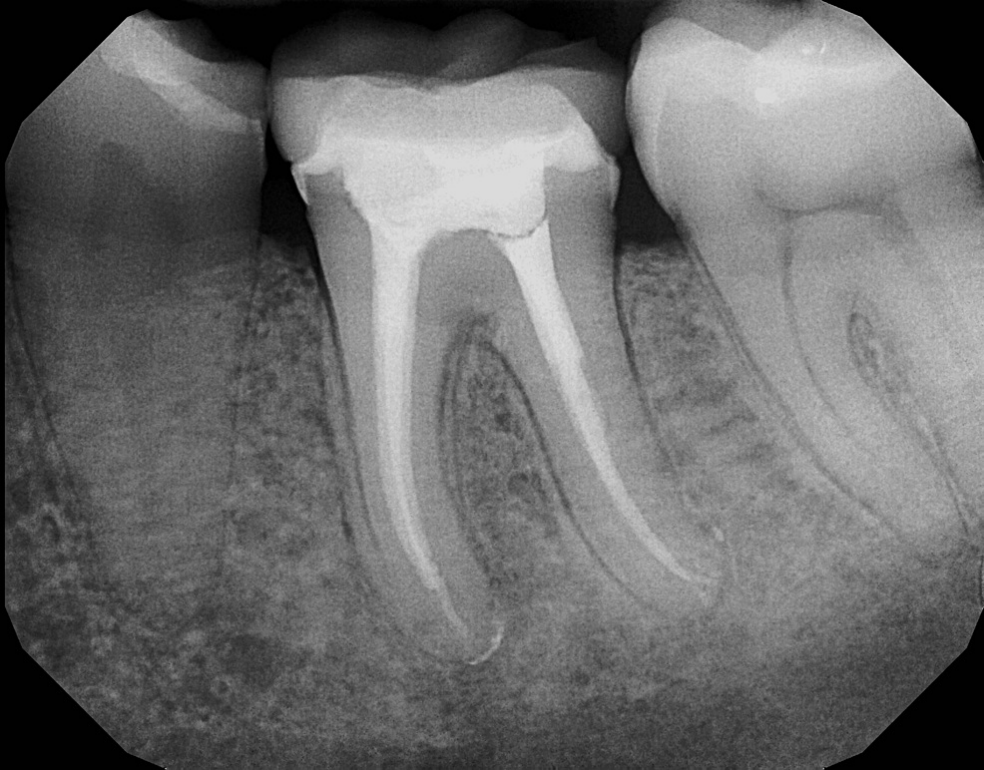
Radiograph of the obturation
